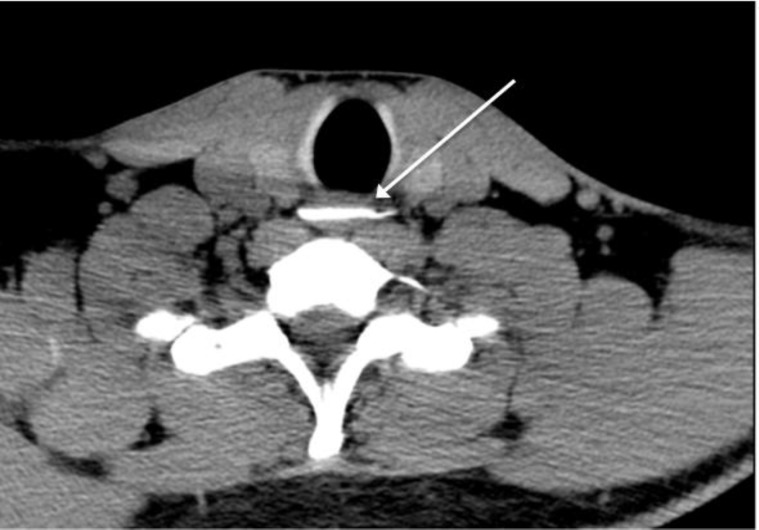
CT-scan: fishbone in the oesophagus, 4 cm below the cricopharynx (case n° 10)
